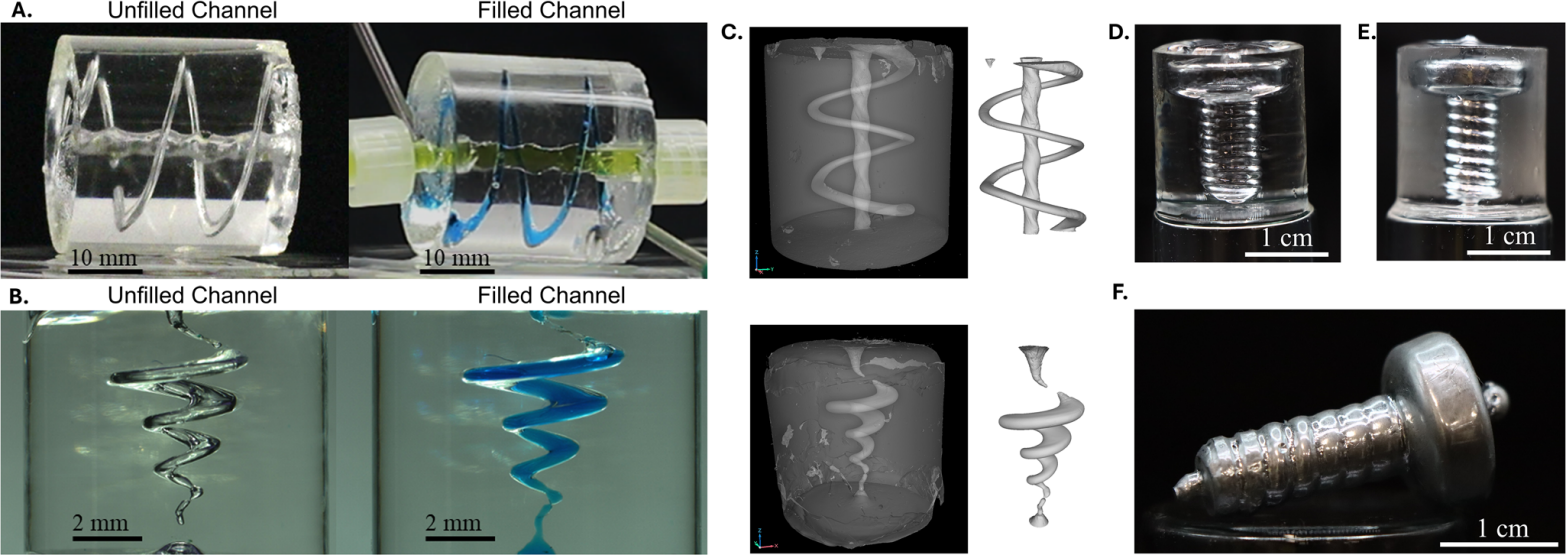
A) T‐VAM printed millifluidic device with and without water dyed with food coloring in the channels (Video S1, Supporting Information). B) Laser‐VAM printed microfluidic device with and without fluid in the channels (Video S2, Supporting Information). C) Images of the CT scans of the millifluidic (top) and the microfluidic (bottom) alongside the channel STLs generated from the CT scans. D) T‐VAM printed instant mold with a screw void in the center. E) Instant mold filled with gallium metal. F) Gallium screw extracted from the instant mold.
